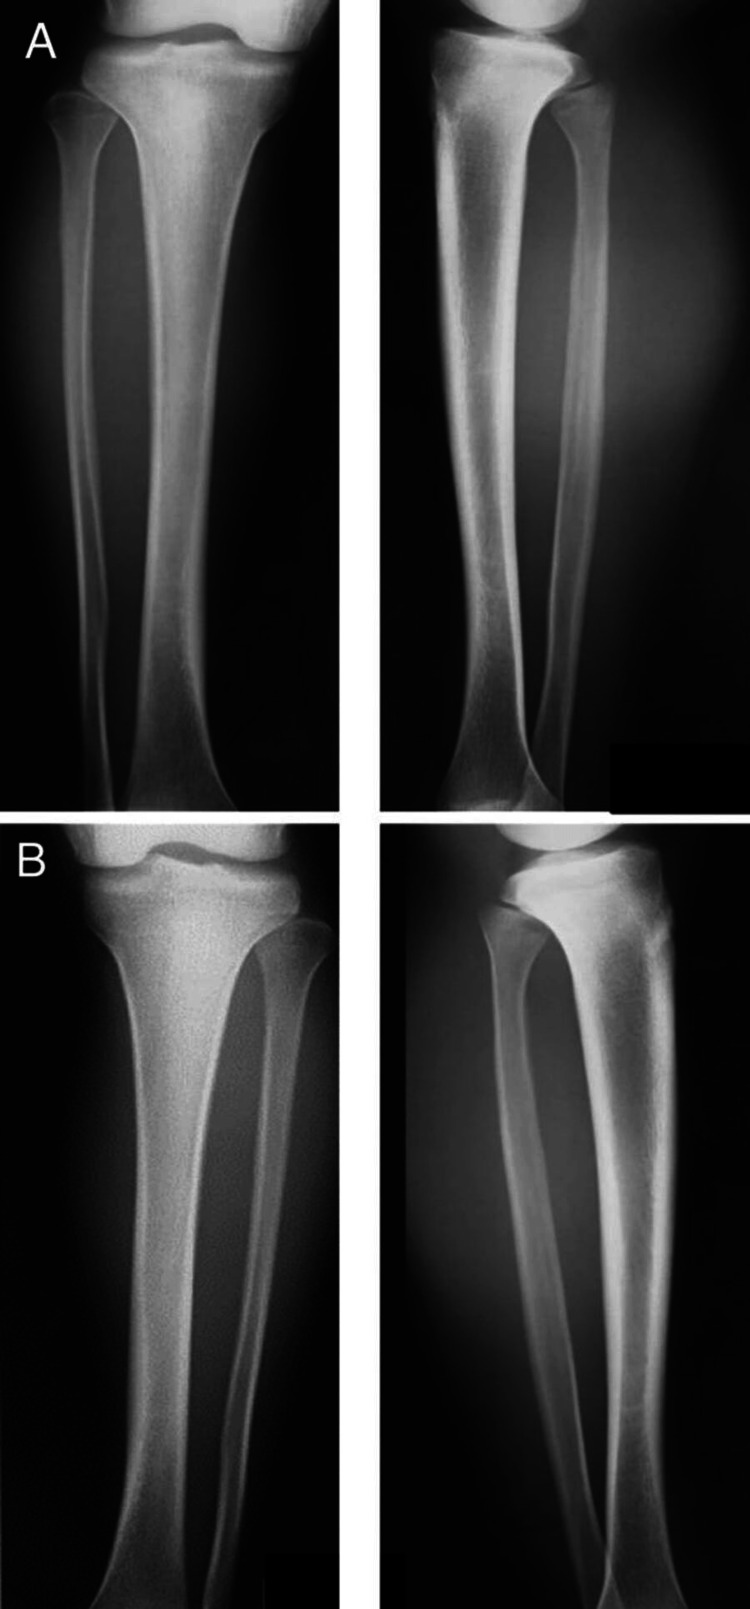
Radiographs of suspected tibial stress fracture no clear abnormality of the tibia in the anteroposterior and lateral views (left): Anteroposterior; (right): Lateral
